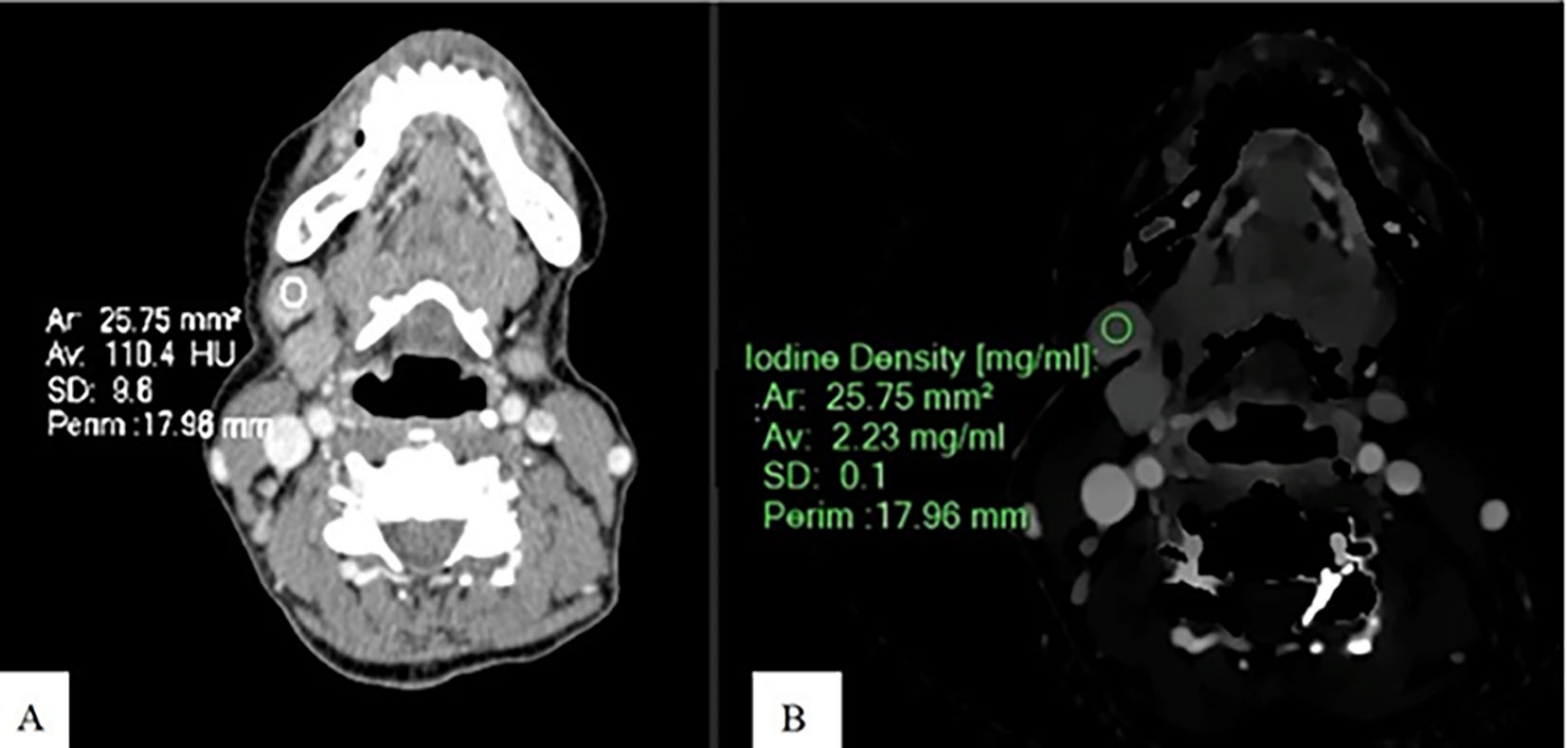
Measurement of ID and CEAV in metastatic SCCA node. An Axial CT image of a patient with SCCA at gum shows CEAV measurement (110.4 HU) of the enlarged lymph node at left cervical level IB (A). An ID measurement (2.23 mg/ml) of this lymph node at the same CT level (B).
